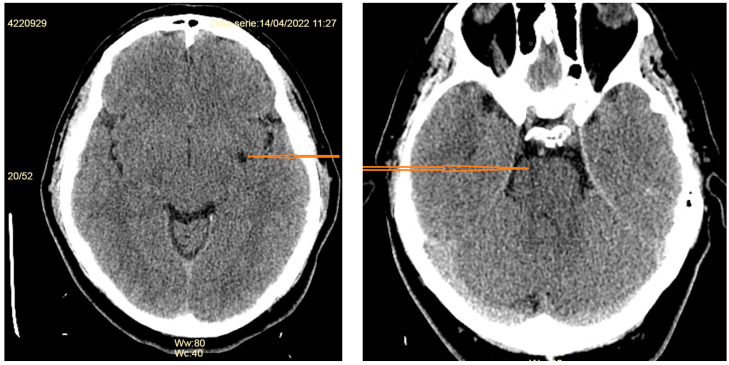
Non-contrast-enhanced CT scan of the patient on admission, showing a lacunar stroke in the left capsulo-lenticular area and the right pons (red arrows).
